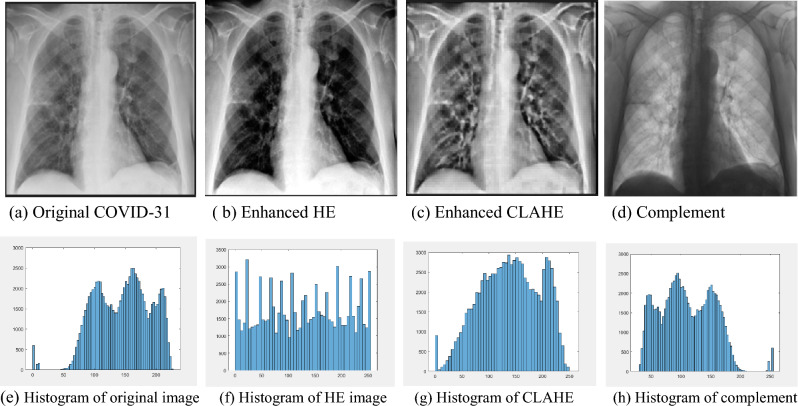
An X-ray image and its enhanced versions after applying HE, CLAHE and complement to the original image and the corresponding histogram plots.
